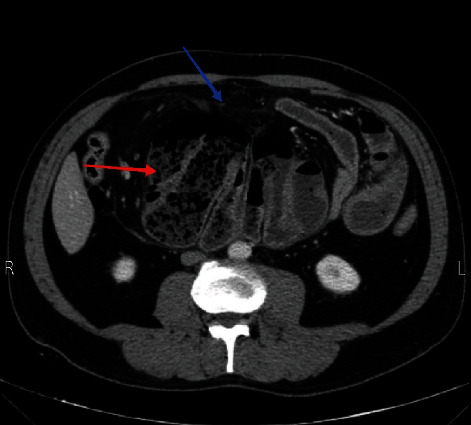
Axial image of contrast enhanced CT shows cluster of small bowel (red arrow) which encapsulated by peritoneum (blue arrow).
